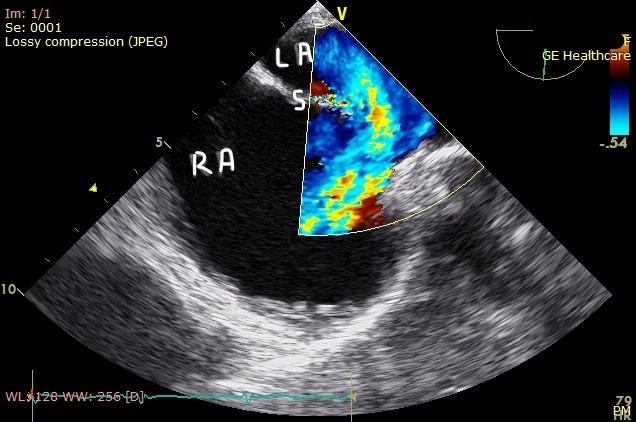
Transesophageal echocardiography showed an atrial septal defect of the superior sinus venosus type with Left to Right flow. RA, right atrium; LA left atrium; S atrium septum with septum defect
